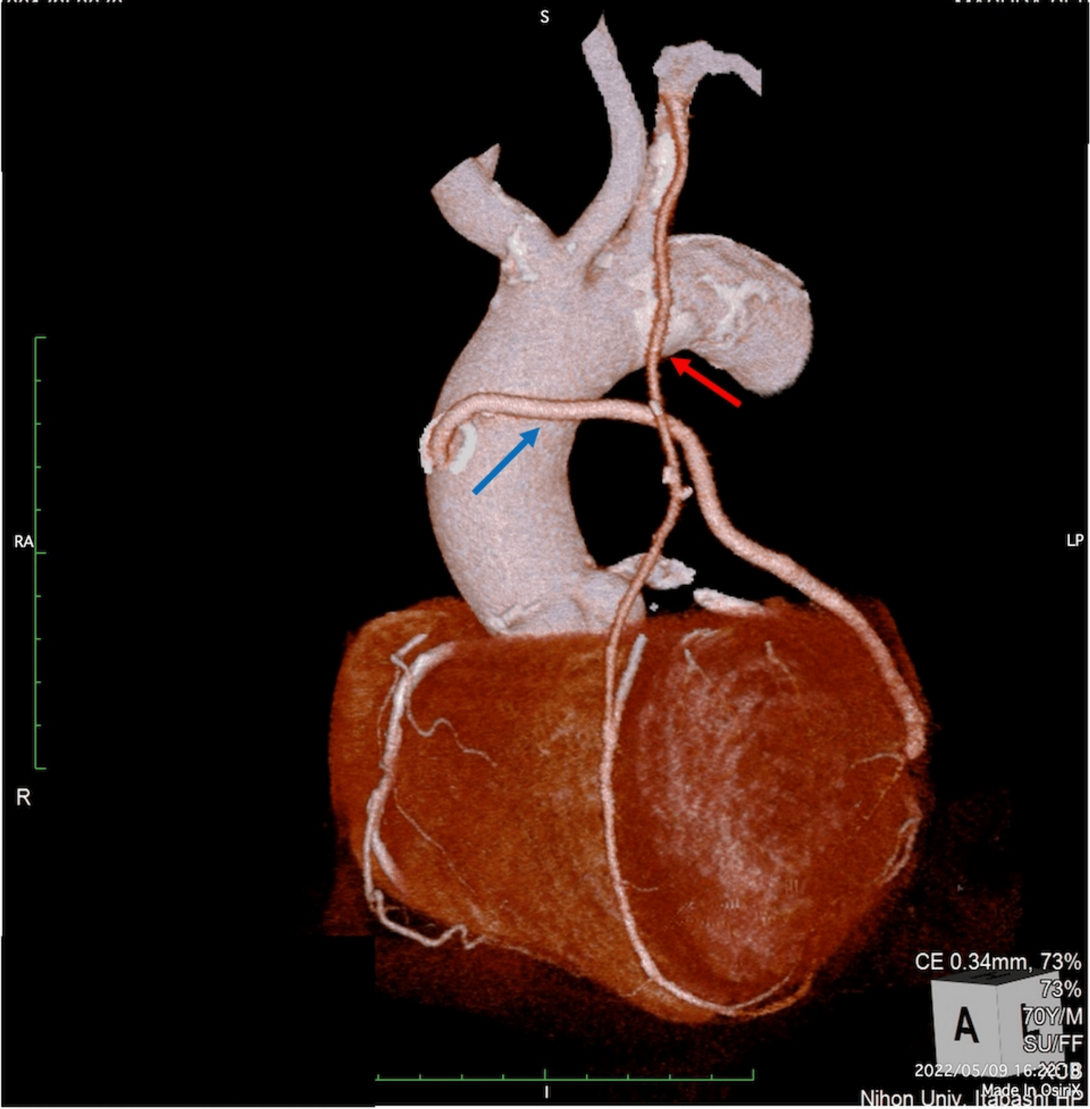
Coronary computed tomography angiography after coronary artery bypass grafting Coronary computed tomography angiography with contrast media showing patent grafts (left internal thoracic artery (red arrow) and saphenous vein (blue arrow))
